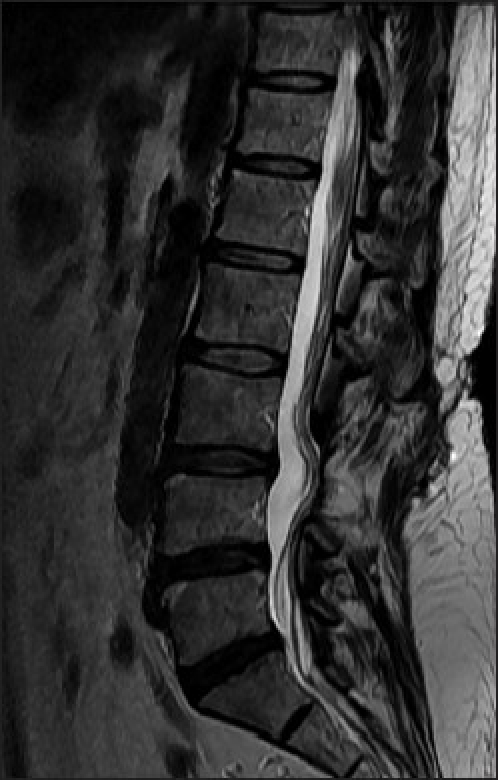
T2-weighted sagittal MRI images at 14-month follow-up after surgery. No recurrent cyst and no evidence of progression of degenerative change in the L3-4 disc were observed
